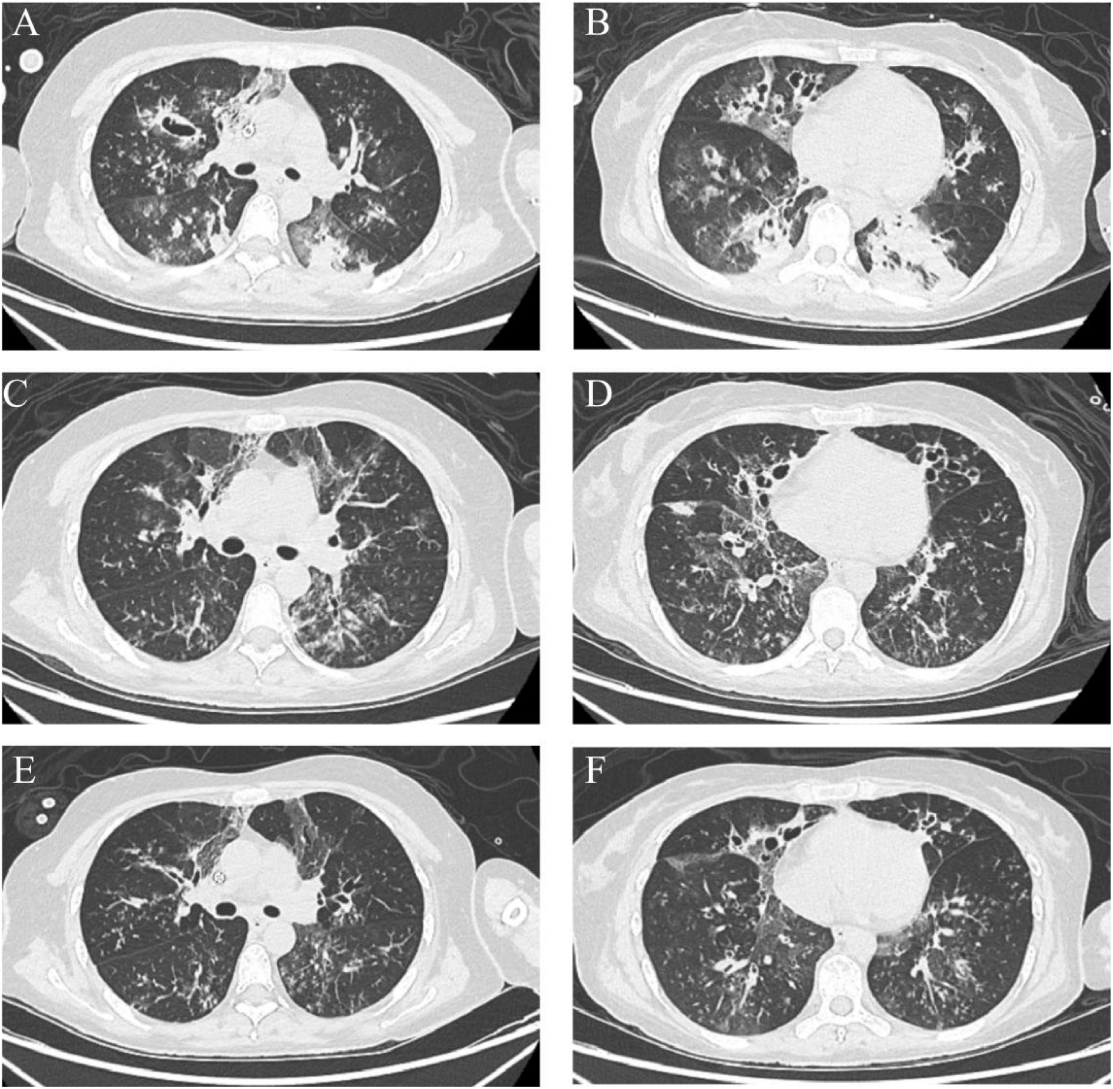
Chest CT showing interval changes in bilateral bronchiectasis. (A,B) Multifocal bilateral cylindrical/cystic bronchiectasis with bronchial wall thickening and surrounding patchy opacities on 20 March 2023. (C,D) Persistent multifocal bronchiectasis with wall thickening and patchy peribronchial opacities, showing interval improvement compared with the prior scan on 12 April 2023. (E,F) Further interval improvement of the peribronchial patchy opacities, with residual bronchiectasis and bronchial wall thickening on 28 April 2023.
